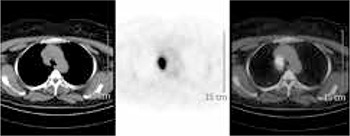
Selected transaxial CT, corresponding PET and fusion images of mediastinum showing FDG uptake in conglomerate nodules at right upper-lower paratracheal lymph nodes.
